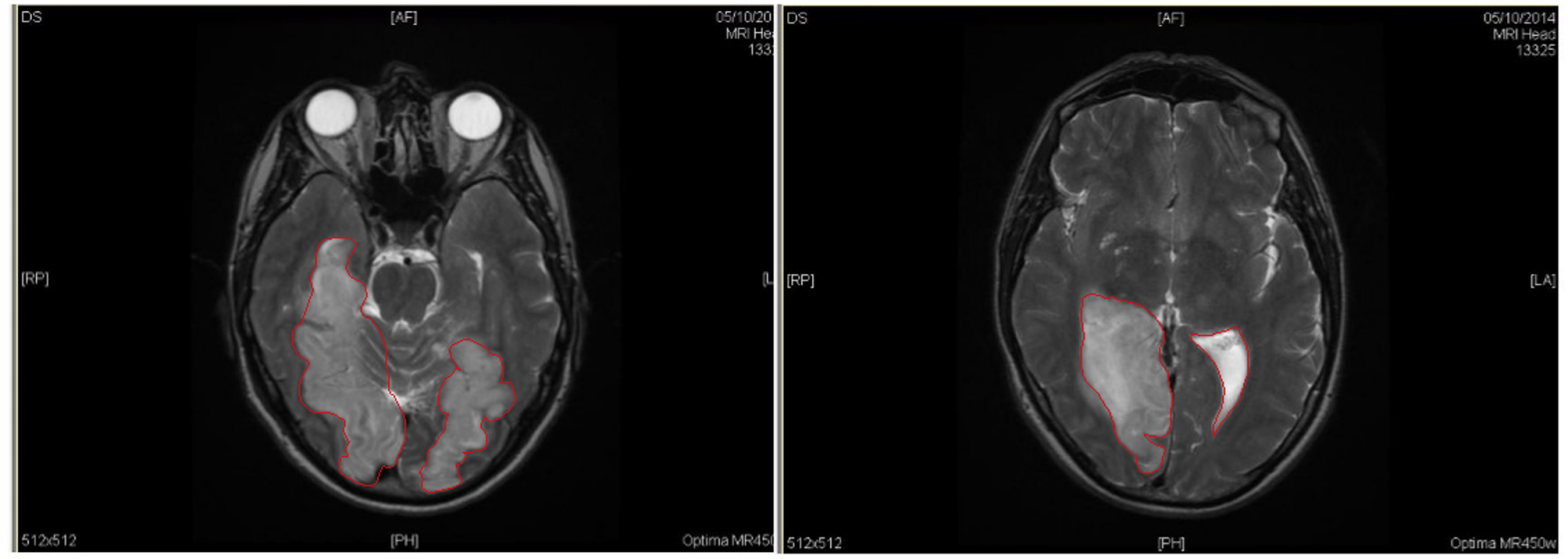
Structural MRI of Patient DS showing the diffuse bilateral brain damage suffered inthe occipital temporal lobes and unilateral damage in the right anterior temporal lobe, following an ischemic stroke (lesion reconstruction in axial view only). *Note.* In axial views, the horizontal orientation is reversed, meaning the left side of the patient’s brain appears on the right side of the image. The diffuse lesion is highlighted approximately within the regions marked in red.
